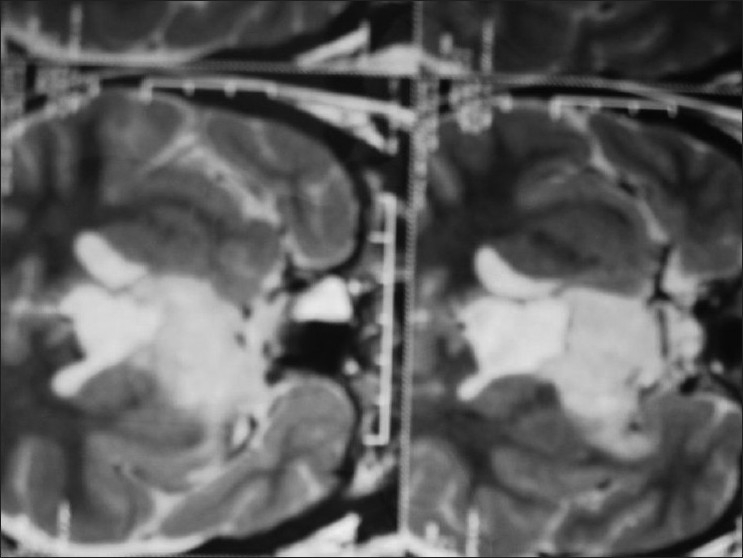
Pre-operative magnetic resonance imaging showing a mass in the third ventricle (3.5 cm × 3 cm × 2.7 cm)
